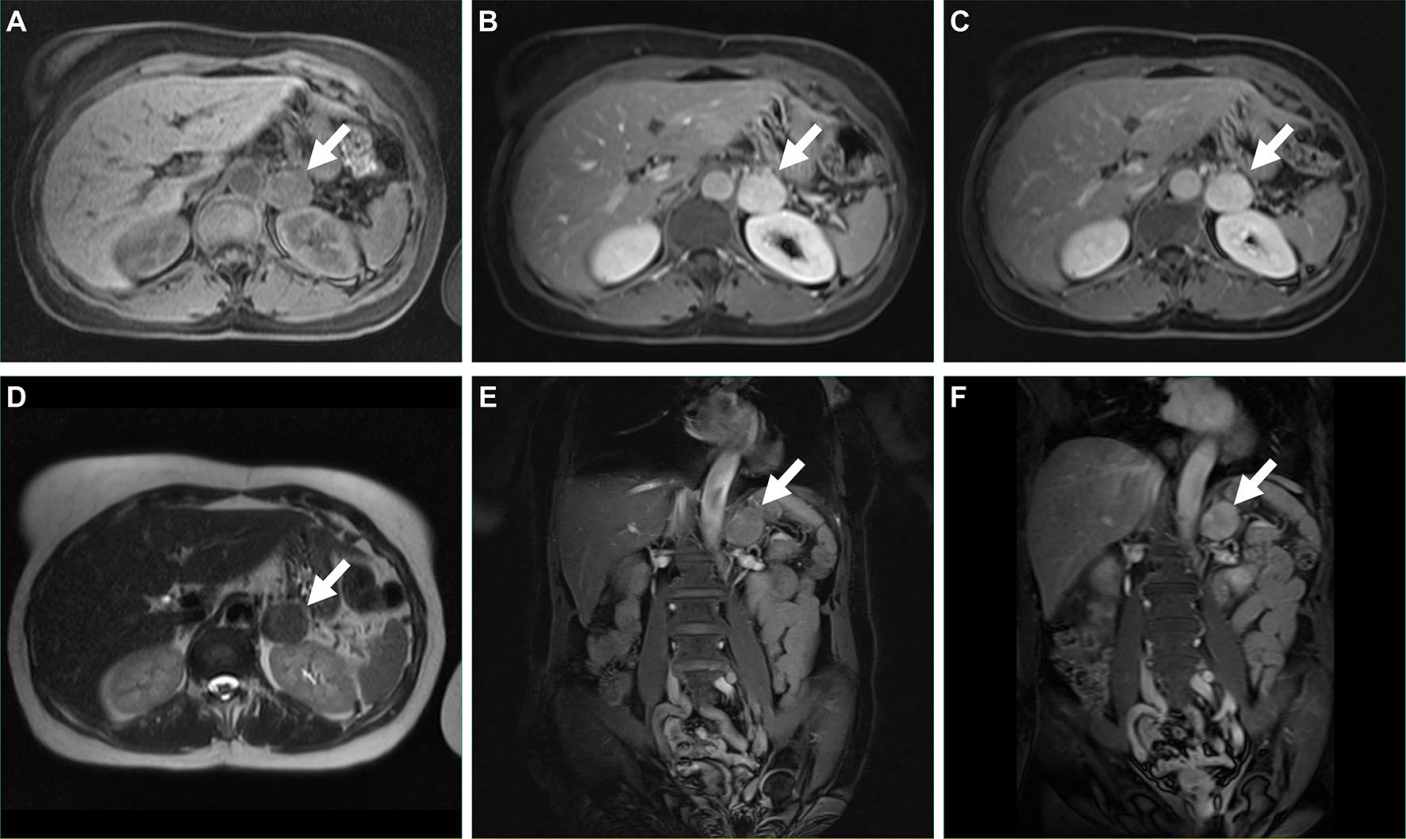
(A–F) MRI of the patient before surgery.
